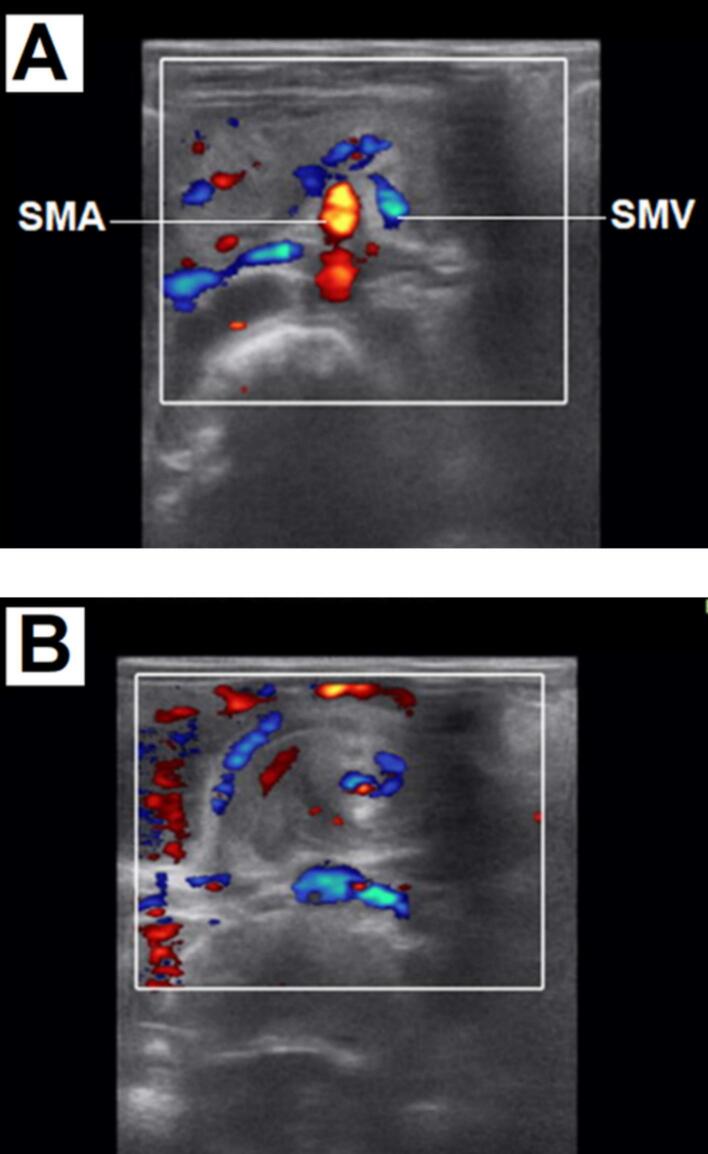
(A) The Color Doppler of upper abdomen shows reversal of SMA-SMV. (B) Color Doppler ultrasound of the upper abdomen shows whirlpool sign.
